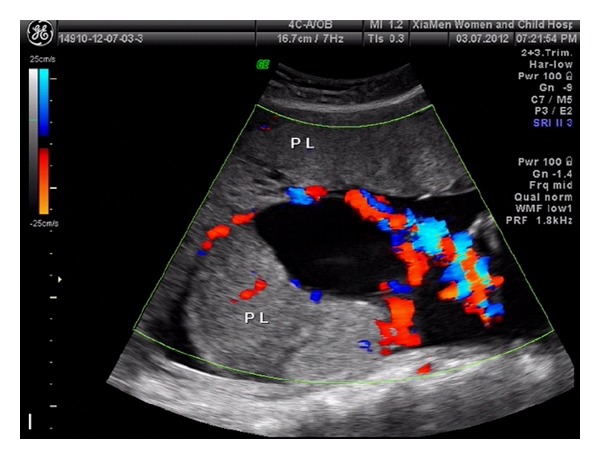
Ultrasonography shows a circumscribed echogenic mass measuring 115 × 110 × 112 mm containing anechoic cystic areas with pulsatile blood flow. The mass protrudes from the fetal surface of the placenta into the amniotic cavity.
